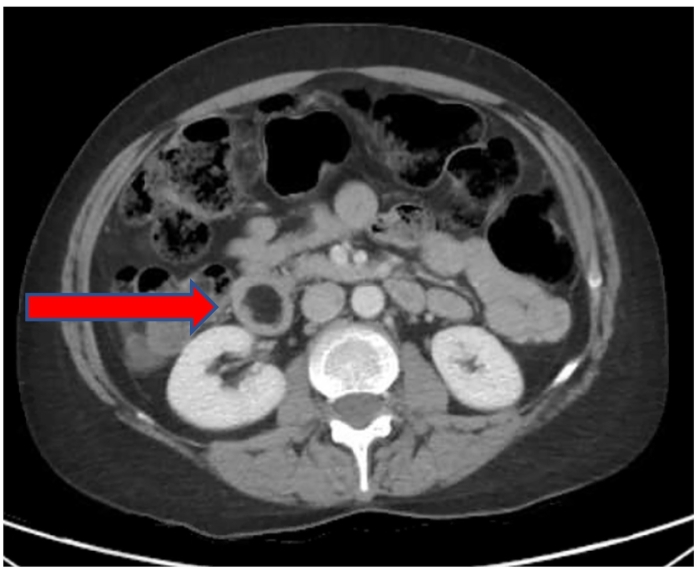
CT scan revealing duodenal mass anterior to right kidney.
